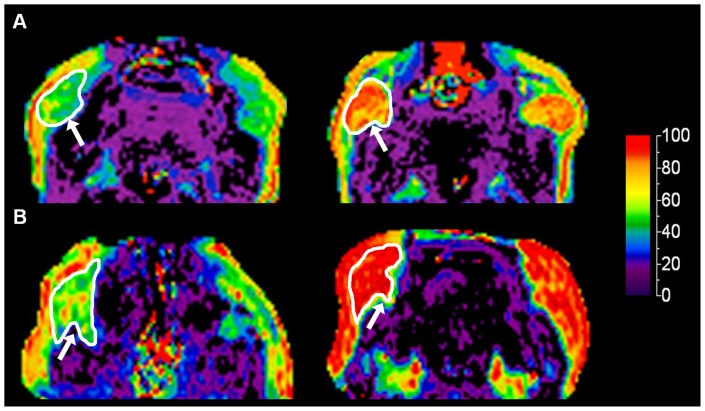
Changes in the composition of the BFP during infancy. Dixon MRI examinations of the BFP (arrow) in a 1 day old male infant (A) and a 6 day old female infant (B) showing increases in FF values from 55 to 84% and 64 to 94% two to six weeks later, respectively.
